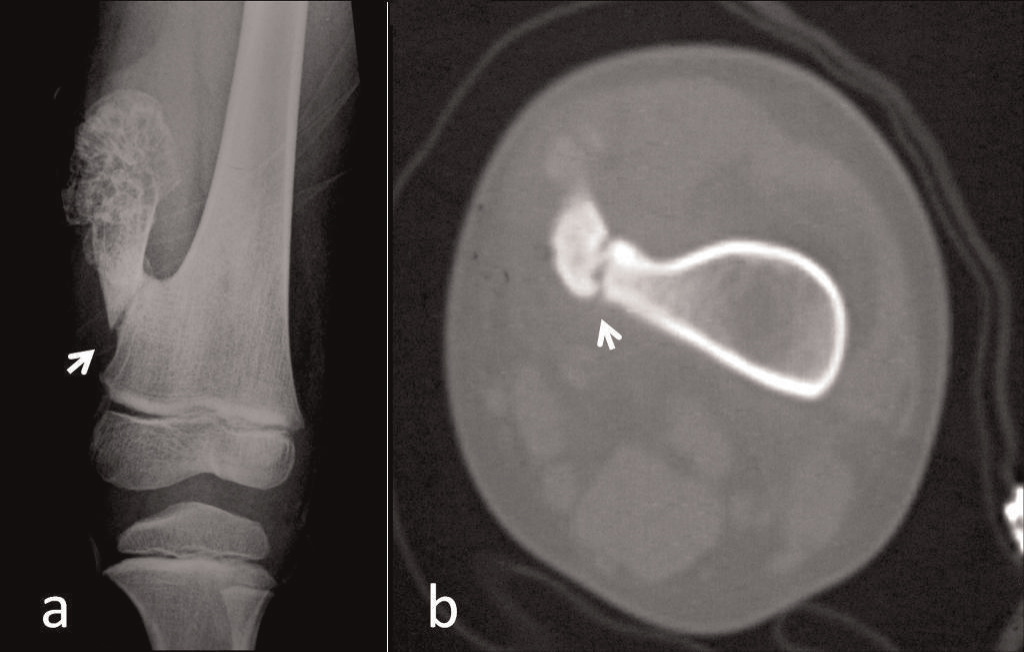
(a) Antero-posterior plain radiograph of the femur. (b) Axial CT scan of the femur through the fracture line. White arrows show the fracture.
